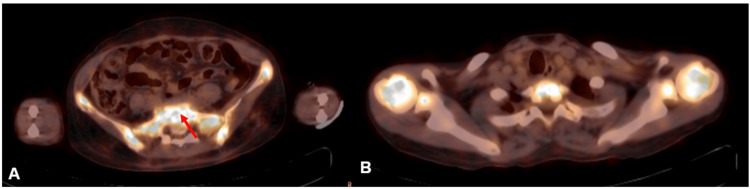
18F-FDG PET-CT showing hypermetabolic sacral involvement in marginal zone lymphoma Axial PET-CT scans showing (A) intense FDG uptake (SUVmax=19.5) in the sacrum (red arrow), consistent with lymphoma-associated skeletal involvement, and (B) heterogeneous FDG uptake in the bilateral humerus and vertebrae, indicating multifocal bone disease. PET-CT: positron emission tomography-computed tomography; FDG: fluorodeoxyglucose; SUVmax: maximum standardized uptake value
